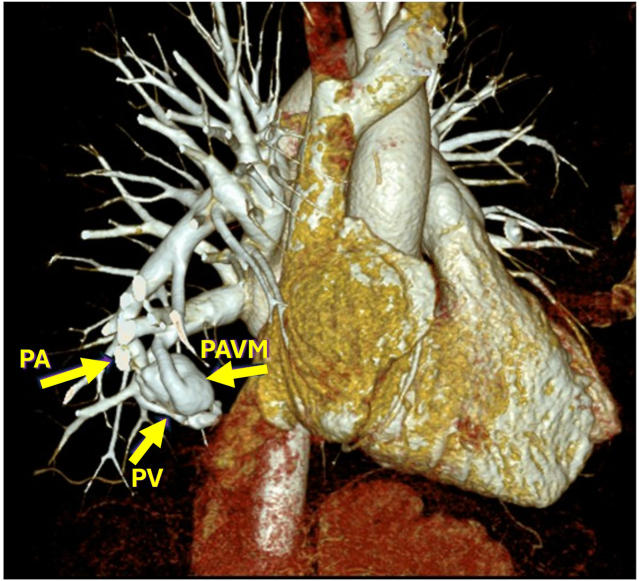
Chest CT angiography, three-dimensional volume-rendered reconstruction, anterior projection, demonstrates a complex PAVM with multiple hypertrophied feeding pulmonary artery branches (PA) and several draining pulmonary veins (PV).
